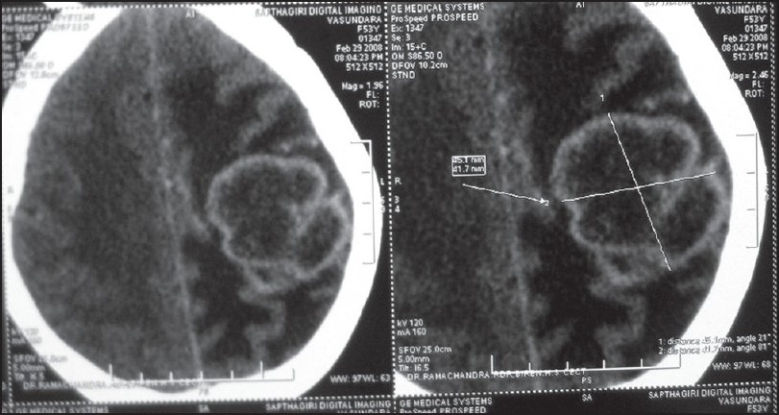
CT scan of the brain shows large left parietal space occupying lesion with perilesional edema
